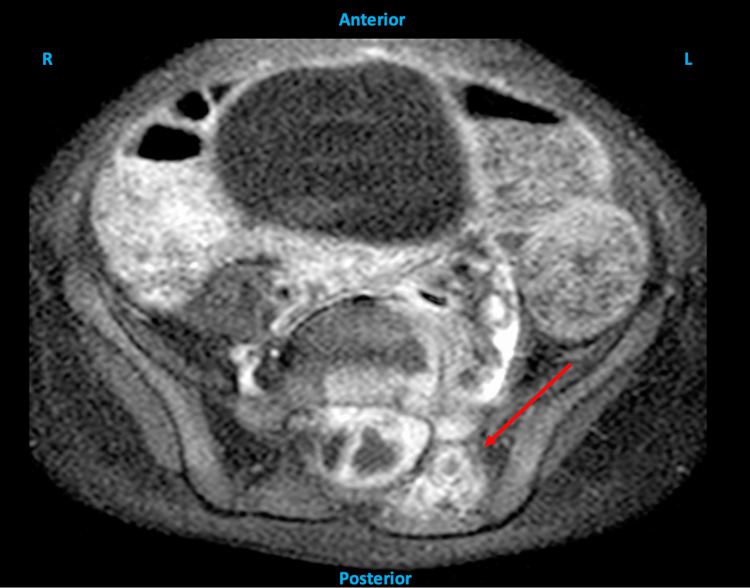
Axial MRI of the lumbar spine showing contrast enhancement in the dorsal paraspinal soft tissues surrounding the left L5-S1 facet joint (red arrow).
